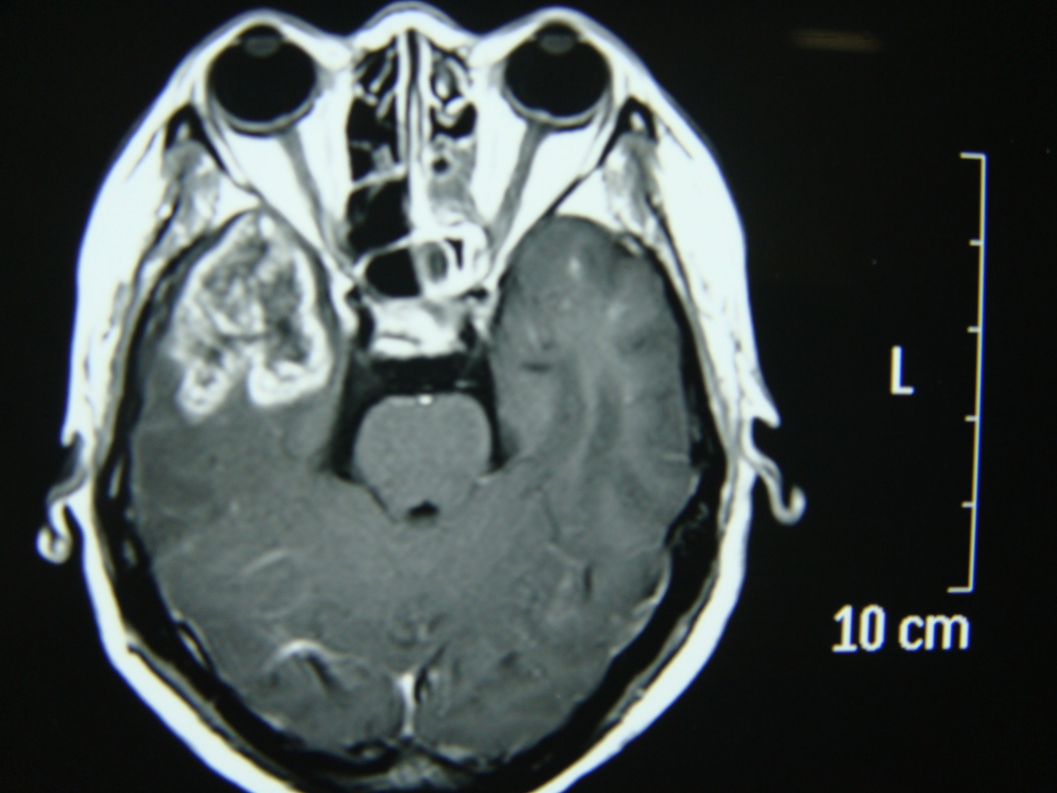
Axial gadolinium-enhanced T1-weighted images revealed a large heterogeneous mass with circumferential rim enhancement surrounded by extensive edema was found in the inferior part of the right temporal lobe. Small homogeneous lesions with limited edema could also be seen in the left temporal lobe.
